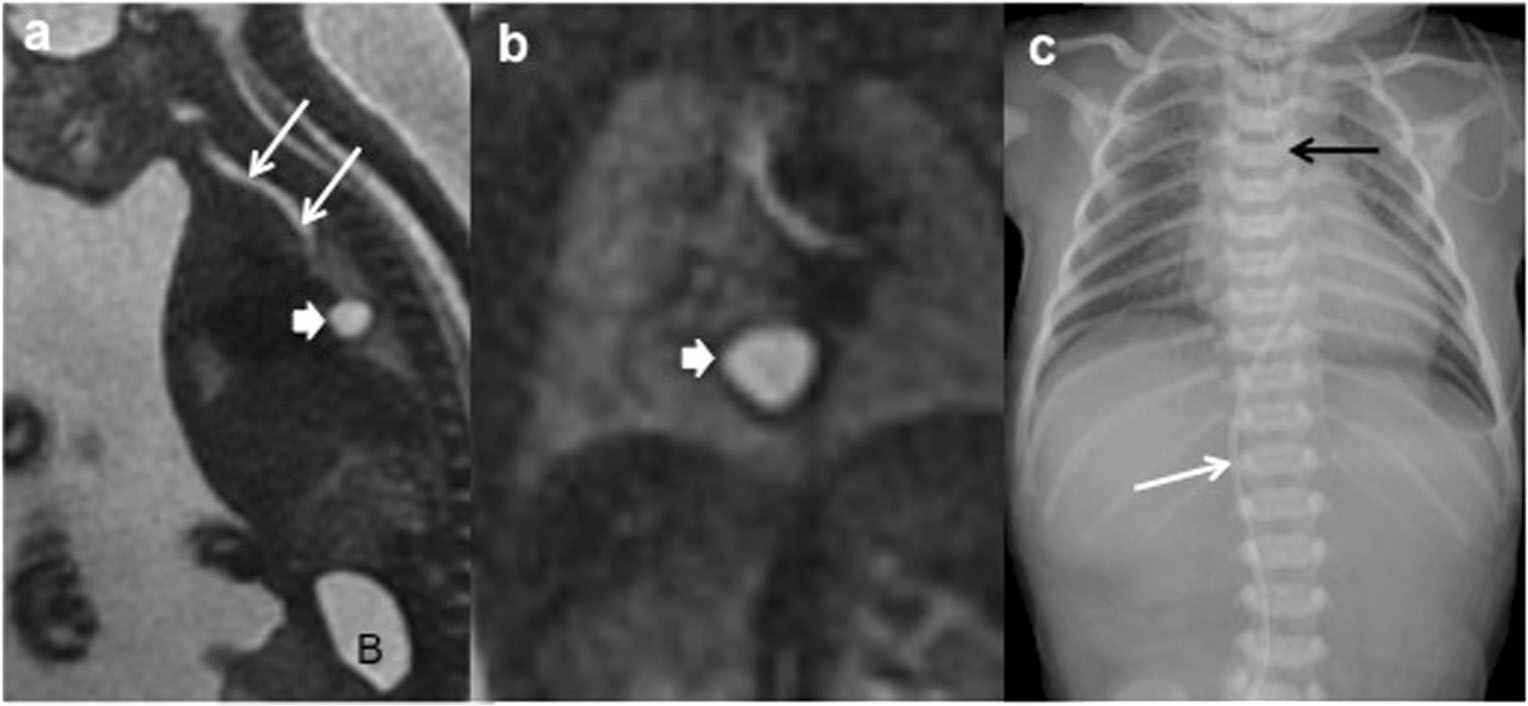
A 21-week-old fetus with polyhydramnios and absent normal gastric bubble in the US is studied. a Sagittal FIESTA fetal MRI showing a mediastinal cyst (thick arrow). Oesophageal atresia without fistula is suspected. *B* bladder. Trachea (*arrows*). b Coronal HASTE fetal MRI: detailed view of the mediastinal cyst (*thick arrow*). c Postnatal thoraco-abdominal radiograph: the gastric line tip is seen (*black arrow*) confirming the oesophageal atresia. The absence of air in the abdomen indicates a type-I or -II oesophageal atresia (without fistula). Venous umbilical catheter (*white arrow*)
